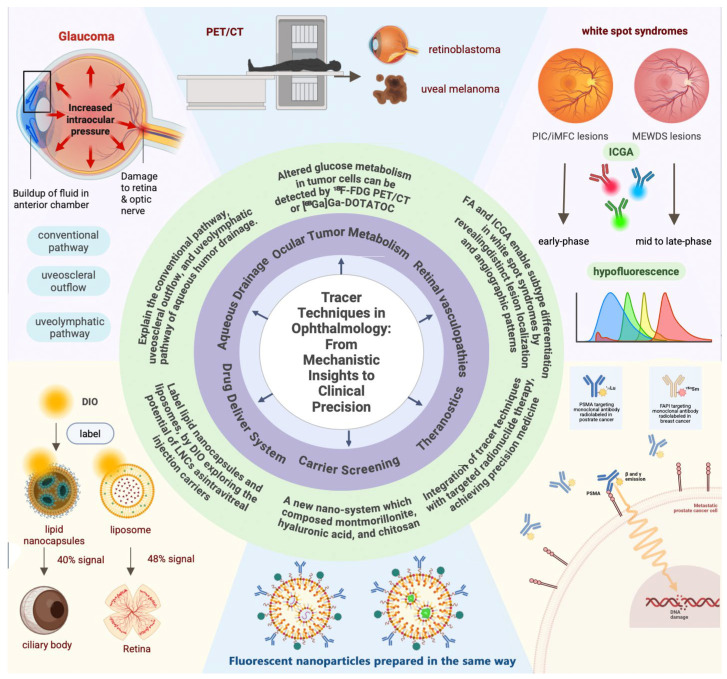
Tracer techniques in ophthalmology: from mechanistic insights to clinical precision PET/CT, positron-emission tomography/computed tomography; FDG, fluorodeoxyglucose; PIC, punctate inner choroidopathy; MC, multifocal choroiditis; MEWDS, multiple evanescent white dot syndrome; ICGA, indocyanine green angiography; FA, fluorescein angiography; PSMA, prostate-specific membrane antigen; FAPI, fibroblast activation protein inhibitor; LNC, lipid nanocarrier.
